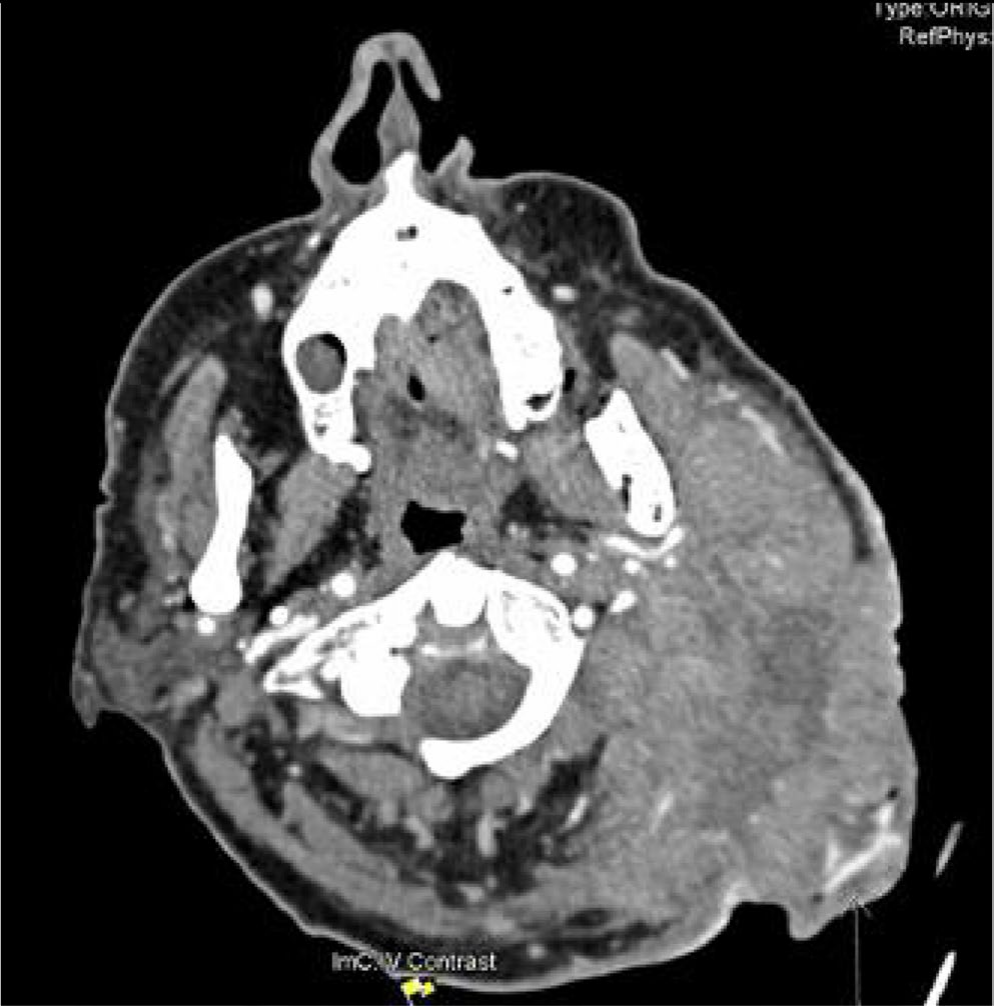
CT neck prior to commencing immunotherapy.
